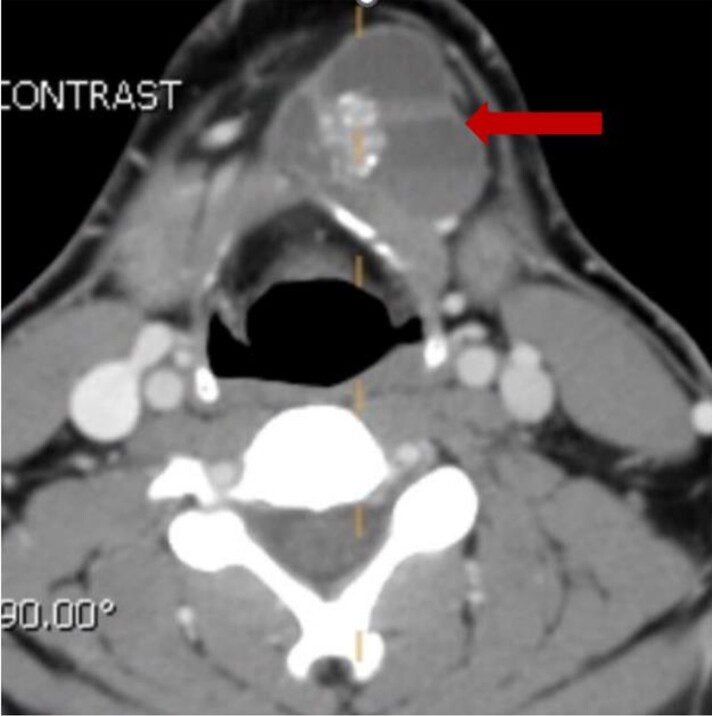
Computed tomography image—axial section showing multiloculated infra hyoid cystic mass with internal punctate calcifications and fluid-fluid levels.
